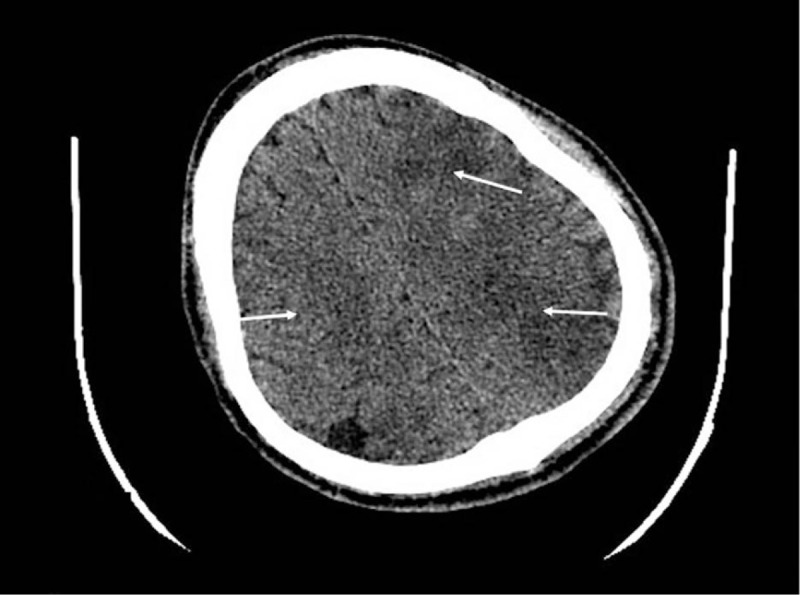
Craniocerebral CT showed multiple infarcts in both frontal and occipital lobes (as shown by the arrow).
